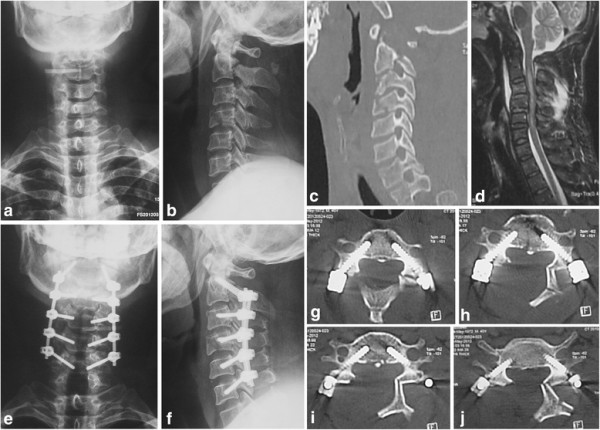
Radiographic studies of the cervical defects and performed procedures. (a–c) Preoperative anteroposterior images of vertebral fracture in cervical vertebrae C2 and C3. (d) Spinal stenosis between C3 and C4, and between C4 and C5 are shown with preoperative magnetic resonance imaging; spinal cord deformation, posterior ligament complex injury, and hematoma anterior to vertebrae are revealed between C2 and C5. (e–f) Open-door posterior laminoplasty and transpedicle internal fixation at C2–C5. (g–j) Transpedicle screws were embedded into the pedicle.
